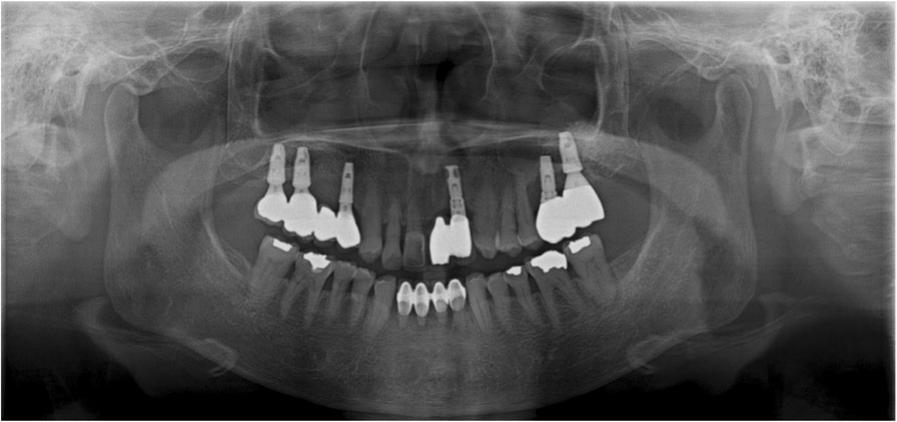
F2, the final panoramic radiograph showing bone loss at #26i and 27i
